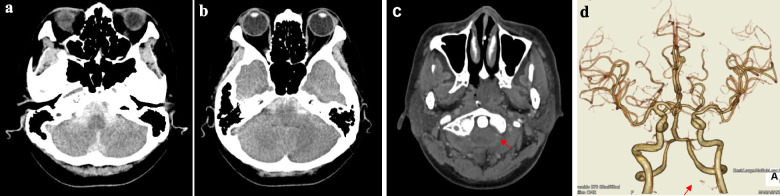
CT scan revealed subarachnoid hemorrhage (SAH) (a, b). CT angiography (CTA) showed a punctate contrast effect (red arrow) on the left anterior surface of the C1 cervical spinal cord. No stenosis or occlusion of the vertebral artery (VA) or posterior inferior cerebellar artery (PICA) was noted (c, d)
